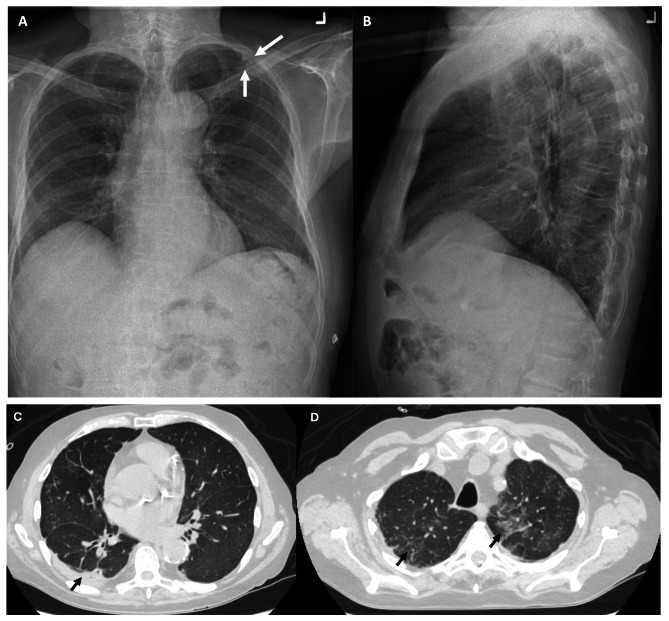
Chest radiography (panel A– PA view, panel B– lateral view) shows dense pleuroparencymal scarring in the left upper lobe (white arrow) that had been present for the preceding six months. CT chest shows progressive bilateral right more than left peripheral consolidation (black arrows) with distortion (panel C). Diffuse bilateral patchy ground glass (black arrows) in the upper lobes were present (panel D)
